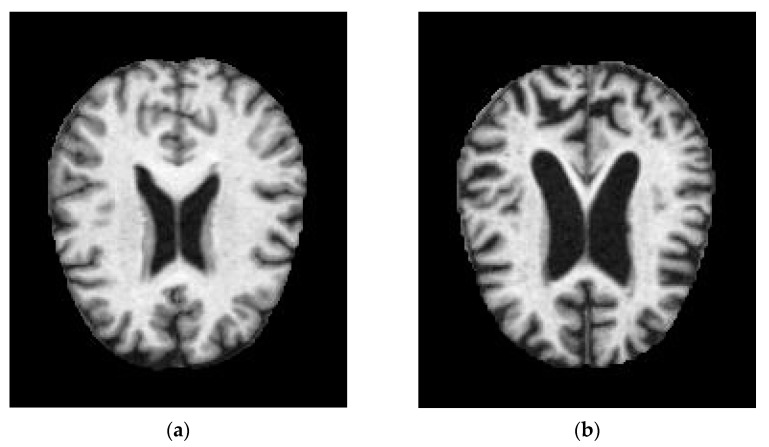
MRI images of (a) normal demented and (b) moderated demented.
